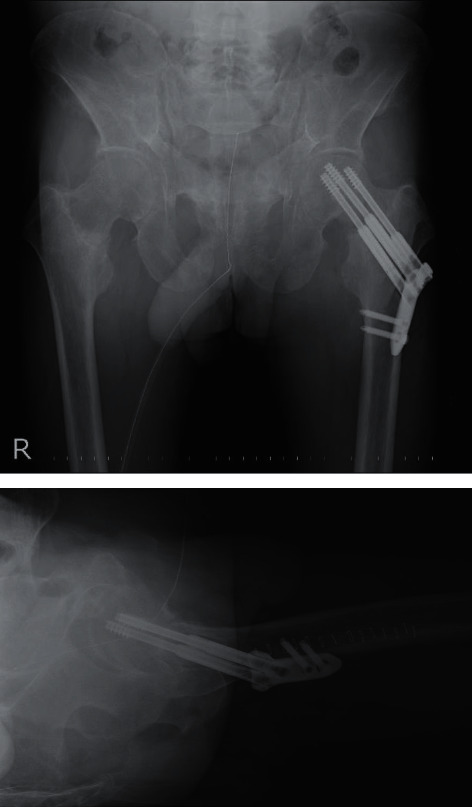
Radiographs taken immediately after surgery. The fracture was fixed using an implant with multiple compression hip screws and a side plate.
